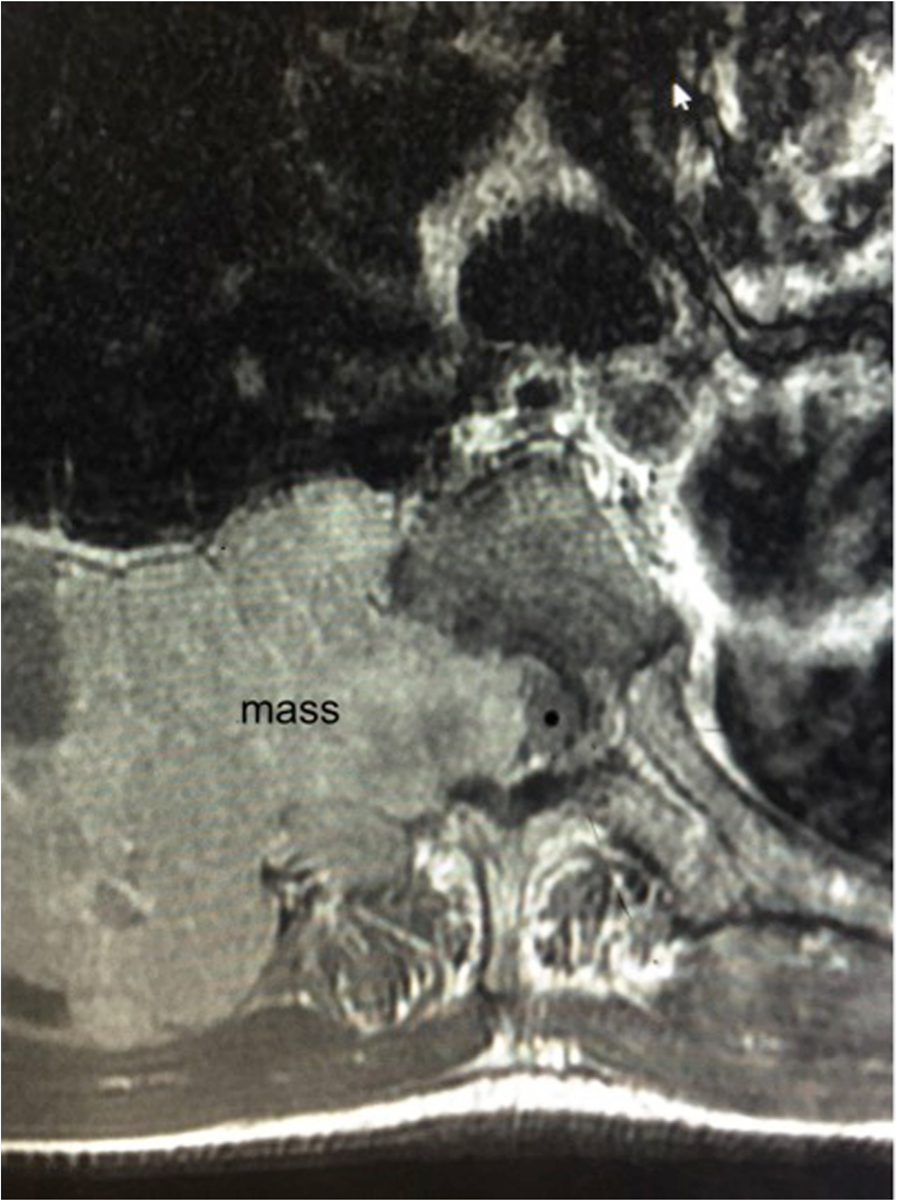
Magnetic resonance imaging of the thoracic spine, sagittal short T1 inversion recovery sequence. Demonstrates metastases to the mid-thoracic spine, T5, T6, and T7, with tumor invasion into the spinal canal and cord compression from T5 to T7
